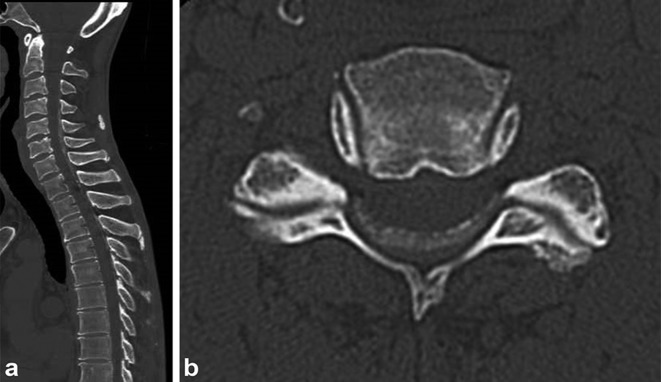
(a) Preoperative CT of the cervicothoracic spine. Sagittal CT shows diffuse epidural calcification at C3-T6. (b) Axial CT of the C5/6 level reveals crescent-shaped mass which has a well-defined border along the vertebral arch.
